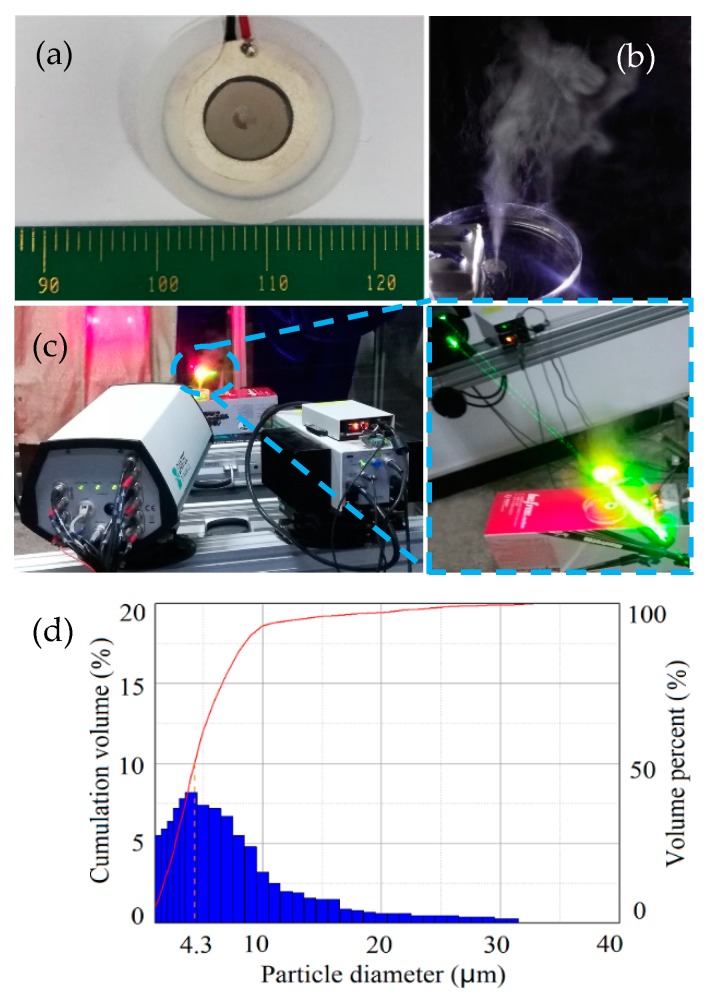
Atomization effect of nebulizer plate and the size distribution of particles using the nebulizer. (a) The prototype of the nebulizer; (b) The atomization effect of the nebulizer; (c) Measuring the nebulizer atomization effect by the phase Doppler particle analyzer (PDPA); (d) The size distribution of particles using the nebulizer.
